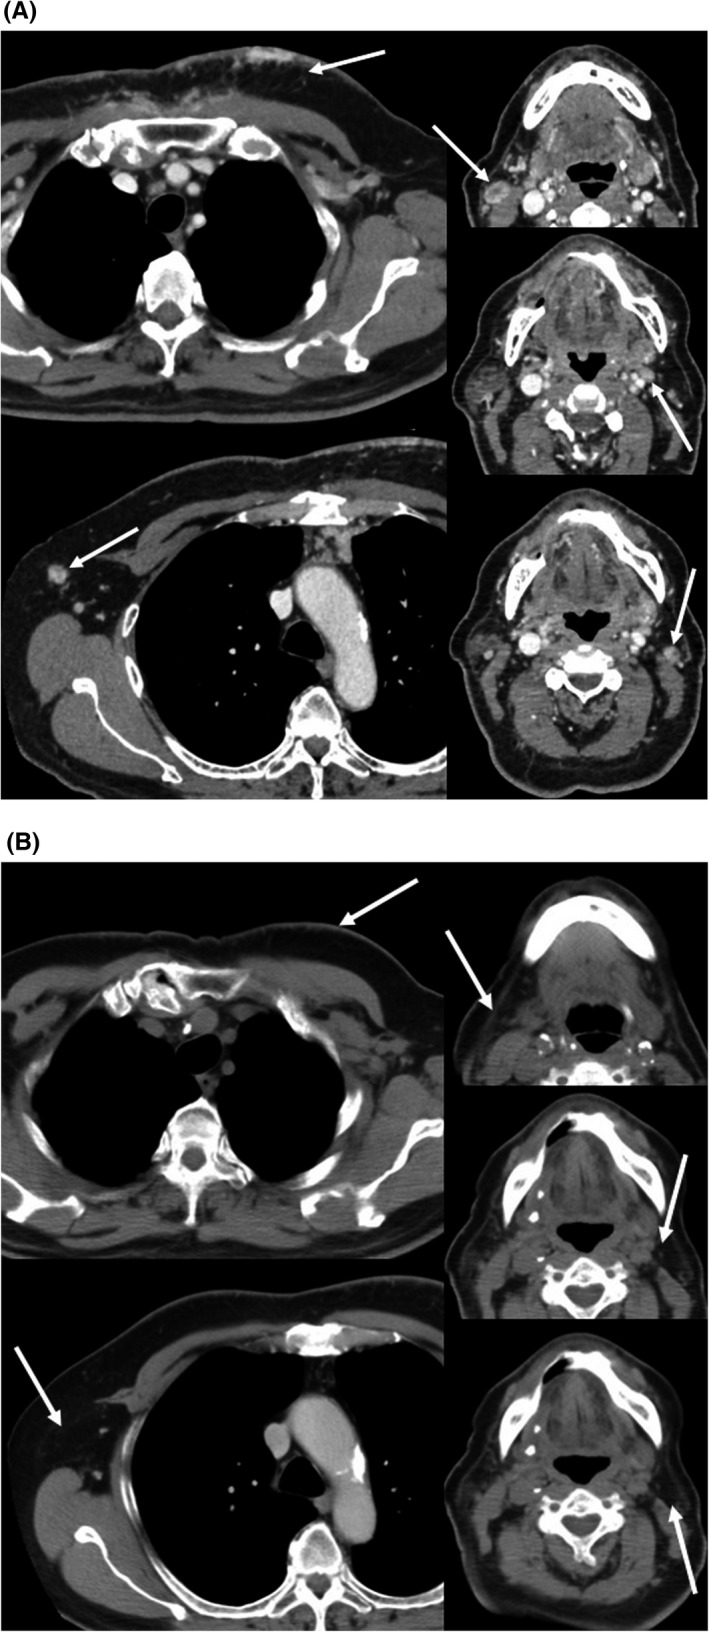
Computed tomography findings. (A) Multiple subcutaneous nodules are visible in the chest (arrow, left upper), axillary lymph node (arrow, left lower), and cervical lymph nodes (arrows, right upper, middle and lower) before he received trastuzumab. (B). The patient exhibited complete response to trastuzumab monotherapy after 5 months (arrows).
